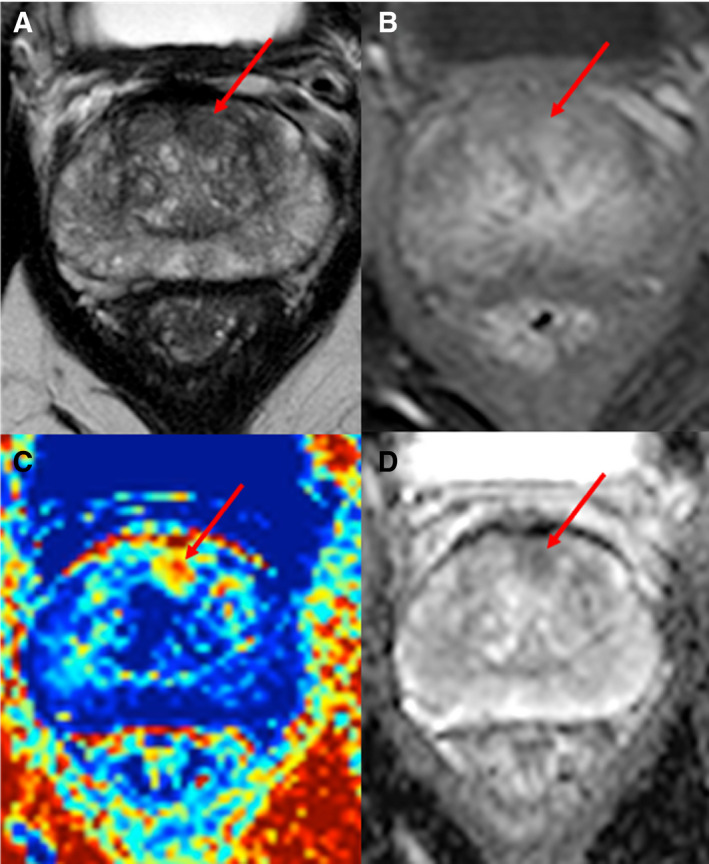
MRI images of a 58 years old with biopsy‐proven PCa. (A) An axial T2‐weighted MRI image showing a focus of homogeneous low signal (red arrow) in the left anterior para‐midline transition zone. (B) T1‐weighted, fat‐suppressed, postcontrast image showing focal enhancement (red arrow) in the left anterior transition zone. (C) VERDICT intracellular volume fraction (FIC) map showing a focal area with increased FIC (red arrow), which enables clearer definition of the biopsy‐positive tumour. (D) ADC map showing reduced signal intensity in the tumour (red arrow).
